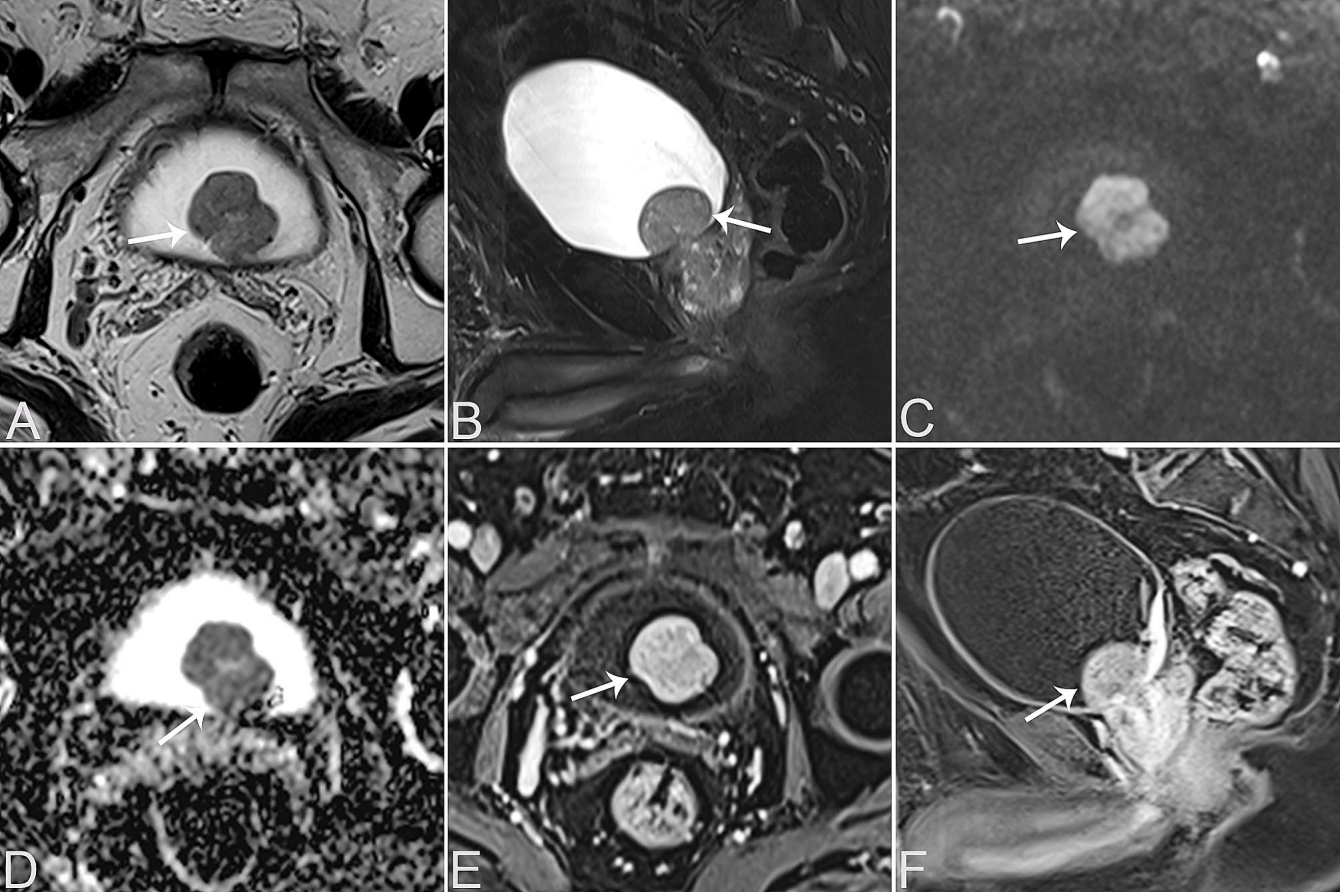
A 74 -year-old male patient with CUC lesion in the bladder neck. Axial T2WI (A) and sagittal T2WI (B) show a well-defined, oval mass in the bladder neck (arrow). The mass appears slightly hyperintense on T2WI. Axial DWI (C) shows the mass with high signal intensity (arrow), and the axial ADC map (D) exhibits marked diffusion restriction (arrow). Contrast-enhanced axial (E) and sagittal (F) T1WI demonstrate the tumor with relatively homogeneous enhancement without apparent necrosis (arrow)
